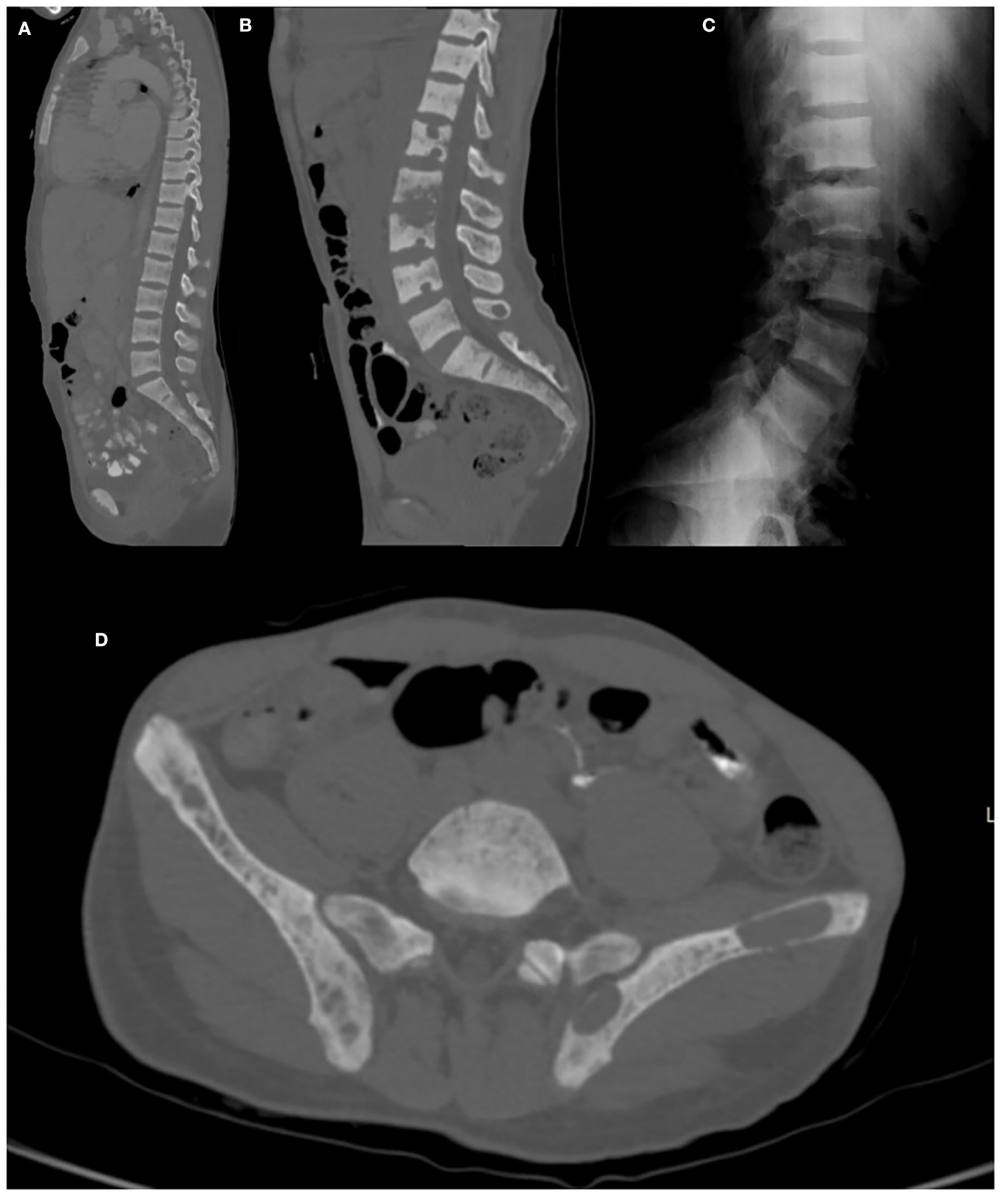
CT sagittal reconstruction of the dorsal and lumbar spine (A,B) of a patient with renal osteodystrophy depicts abnormal bone turnover and mineralization, with diffuse osteosclerosis and multiple areas of subperiosteal resorption. Lateral lumbar spine radiography (C) shows the characteristic “rugger jersey” spine, with alternating bands of increased and normal bone density of the vertebral bodies. Note a large brown tumor of the left iliac bone on CT (D).
